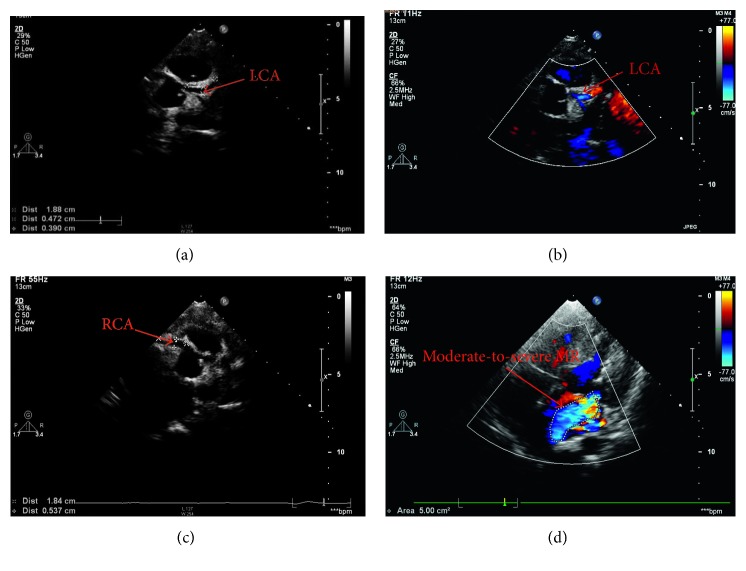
(a, b) The dilated left coronary artery connected to the aorta in the two-dimensional and color doppler flow imaging(CDFI). (c) The dilated right coronary artery connected to the aorta. (d) Moderate-to-severe mitral regurgitation.
